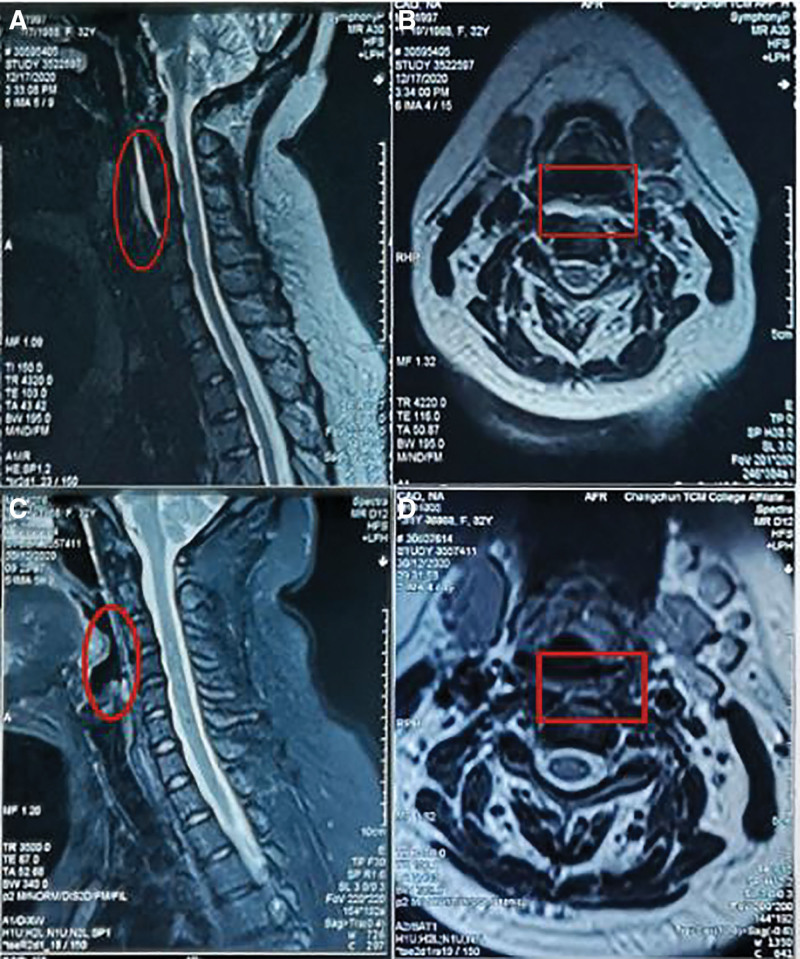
MRI of the cervical spine before acupuncture treatment: (A) cervical spine MRI (sagittal position); (B) cervical MRI (cross section). MRI of the cervical spine after acupuncture treatment: (C) cervical spine MRI (sagittal position); (d) cervical MRI (cross section). MRI = magnetic resonance imaging.
